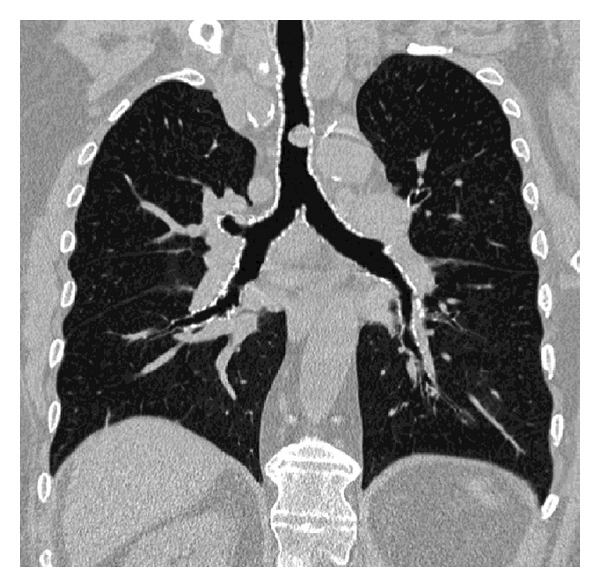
CT scan. CT coronal reformation shows the longitudinal extent of the tumor, its location 35 mm above the carina, and the severity of tracheal narrowing (40%).
